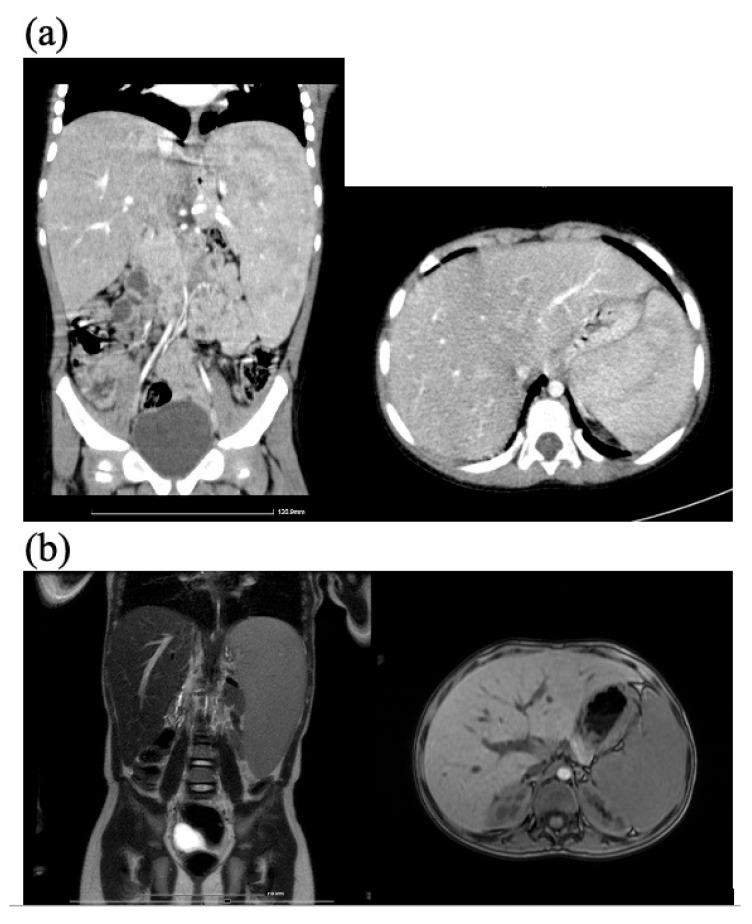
Hepatic and splenic volume evolution in NPD-A/B male: (a) abdominal CT scan at 4 years 7 months—liver volume 737.7 cm^3^, spleen volume 339.13 cm^3^; (b) abdominal MRI scan at 6 years 5 months—liver volume 892.66 cm^3^, spleen 486.11 cm^3^ (images from author’s personal archive).
